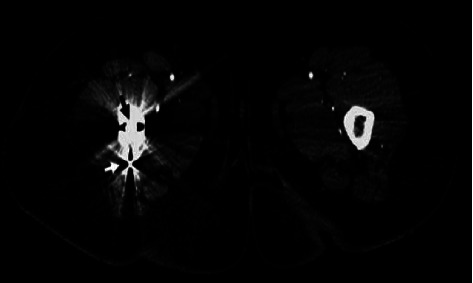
CT with contrast showing resolution of the pseudoaneurysm after embolization (done after 6 months).
